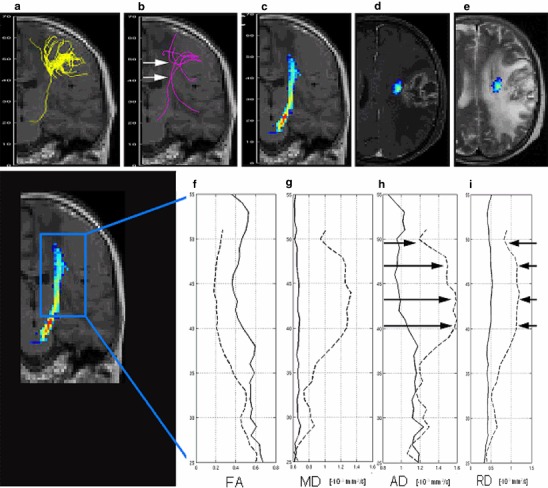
Patient #7 with a *left* frontal glioma with marked motor impairment. FACT algorithm (a) and GT (b) failed in the depiction of the CST, whereas probabilistic maps of connectivity (c) could depict it. Fibres of FACT and GT are given as projections, probabilistic maps of connectivity as cross section. Plots display the values of *FA* fractional anisotropy (f), *MD* mean (g), *AD* axial (h) and *RD* radial diffusivity (i) (given in *n* × 10^−3^ mm^2^/s), obtained from the CST on the pathologic (*dotted line*) and contralateral side (*solid line*) from the *top* (*z* = 55 mm) to the *bottom* (*z* = 25 mm). All diffusivities were increased at the level between *z* = 40–48 mm (*arrows* in b, h and i). Transversal contrast enhanced T1-weighted (d) and T2-weighted images (e) at this level showed a contrast-enhancing tumour in the direct vicinity of the CST and a high signal on T2-weighted images. Both, the tumour infiltration and the vasogenic oedema cannot be distinguished from each other
